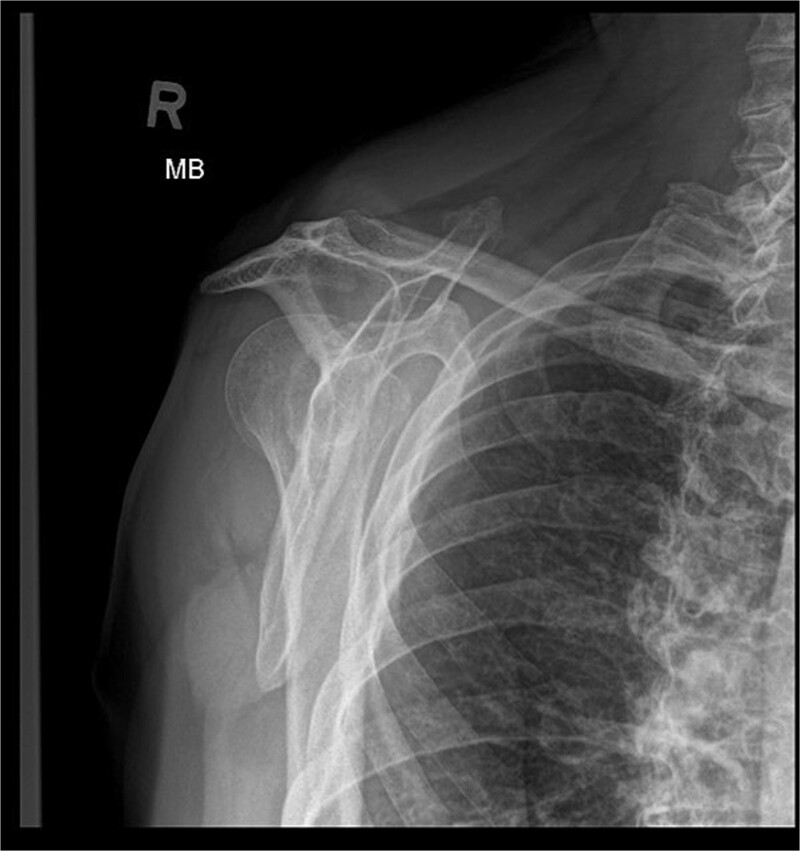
X-ray image before the surgery—Y projection.
